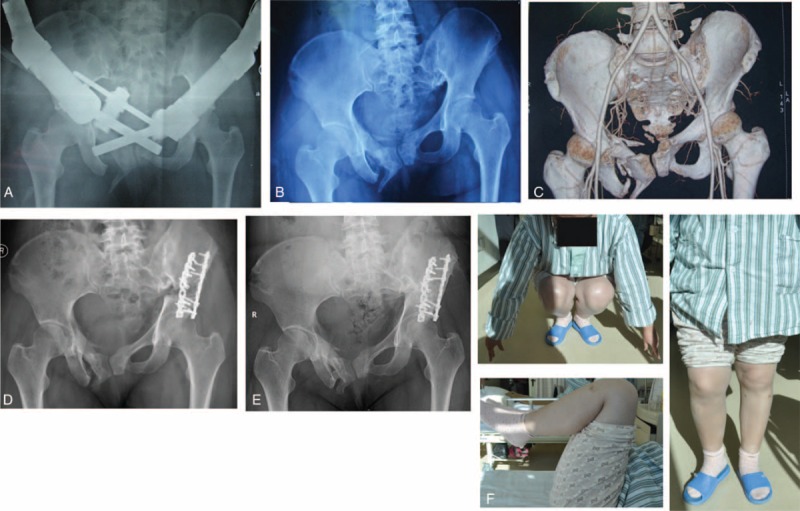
A representative pelvis fracture malunion. A, External fixation after damage control interventions were performed. B, Preoperative anteroposterior X-ray. C, Preoperative 3-dimensional computed tomography reconstruction. D, Anteroposterior X-ray after osteotomy. E, The lower limb function (2 years after surgery). F, The patient's function after the operation.
